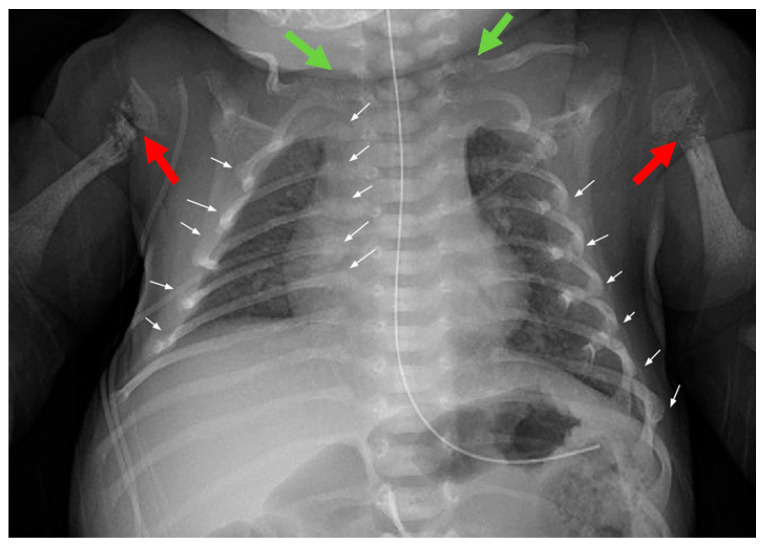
The chest X-ray at 7 days of age showed bilateral fractures of the clavicular heads (green arrows) and likely multiple posterior rib fractures, including the right 2nd–5th ribs, the right lateral 2nd–7th ribs, and the left 3rd–9th ribs (white arrows). Multiple fractures involved the bilateral proximal humeral diaphyses with poorly formed callus at the fracture margins and impaction of the fractures (red arrows). Holick MF, copyright 2021.
